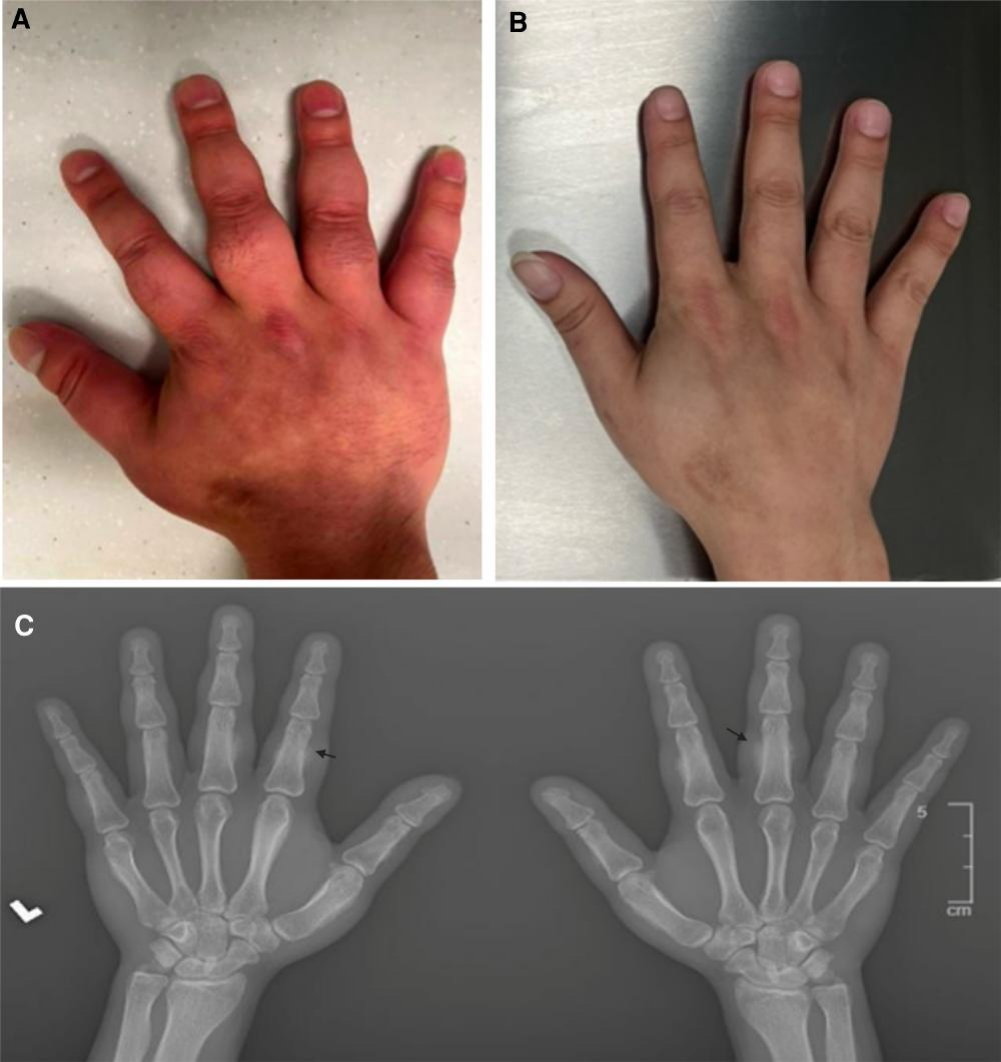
Graves’ acropachy with fusiform swelling (A) improved after teprotumumab (B). X-ray hands (C) showed soft tissue swelling and extensive bilateral periostitis and subperiosteal bone formation (arrows) in multiple metacarpals and phalanges.
